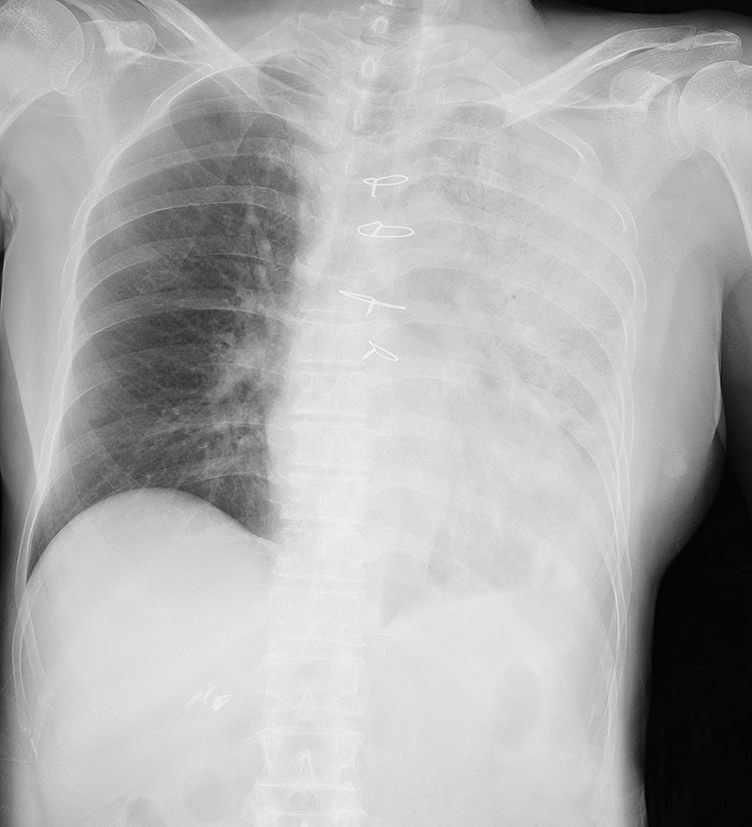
Chest radiography on admission revealed pneumonia over the left lung.
